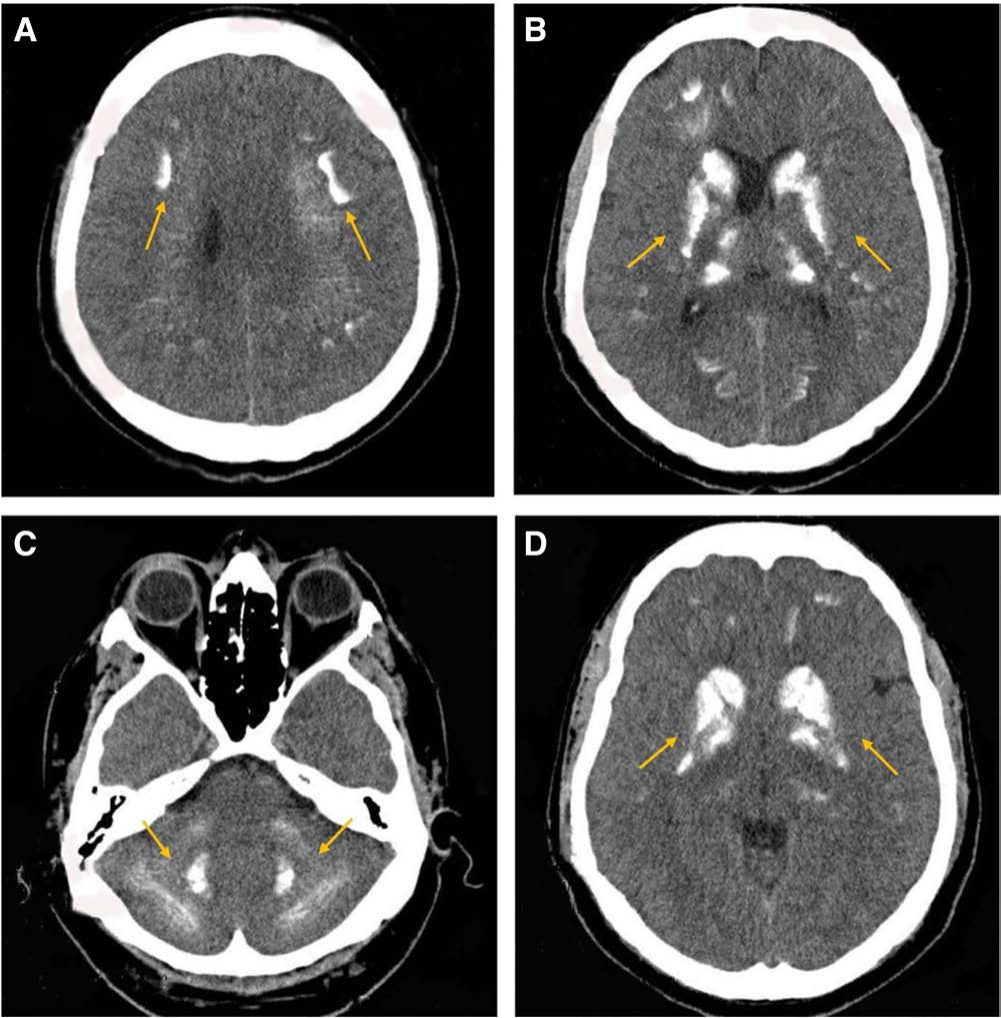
Noncontract CT of the brain showing extensive bilateral symmetric calcification areas in basal ganglia, thalamus, dentate nuclei, and subcortical white matter in axial sections. Arrows show calcification areas.
